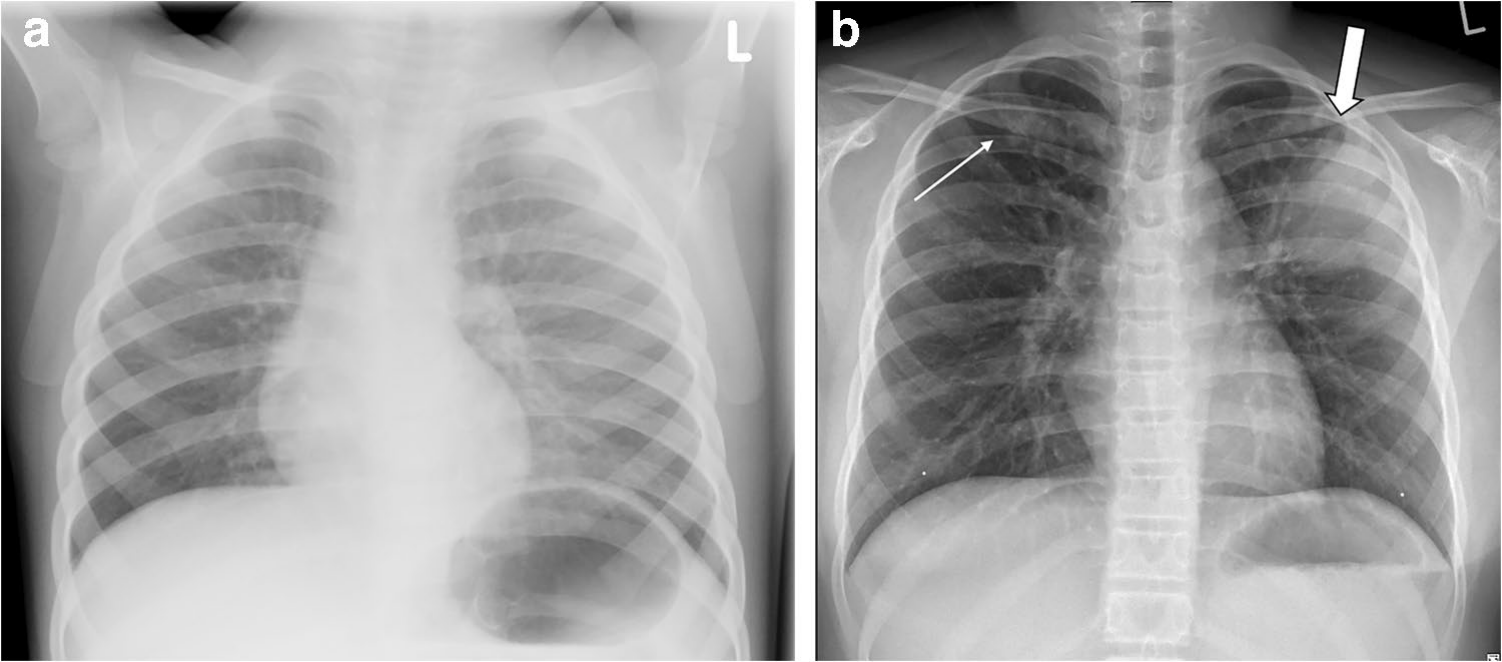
Chest radiography in children with cystic fibrosis and acute pulmonary exacerbation. a, b Anteroposterior (AP) chest radiograph in a 3-year-old boy (a) and posteroanterior (PA) chest radiograph in a 10-year-old boy (b). Note absence of clear abnormalities in (a). Conversely, (b) shows small peribronchial opacity in the right upper lung (*thin arrow*) and large consolidation in left upper lung (*thick arrow*)
